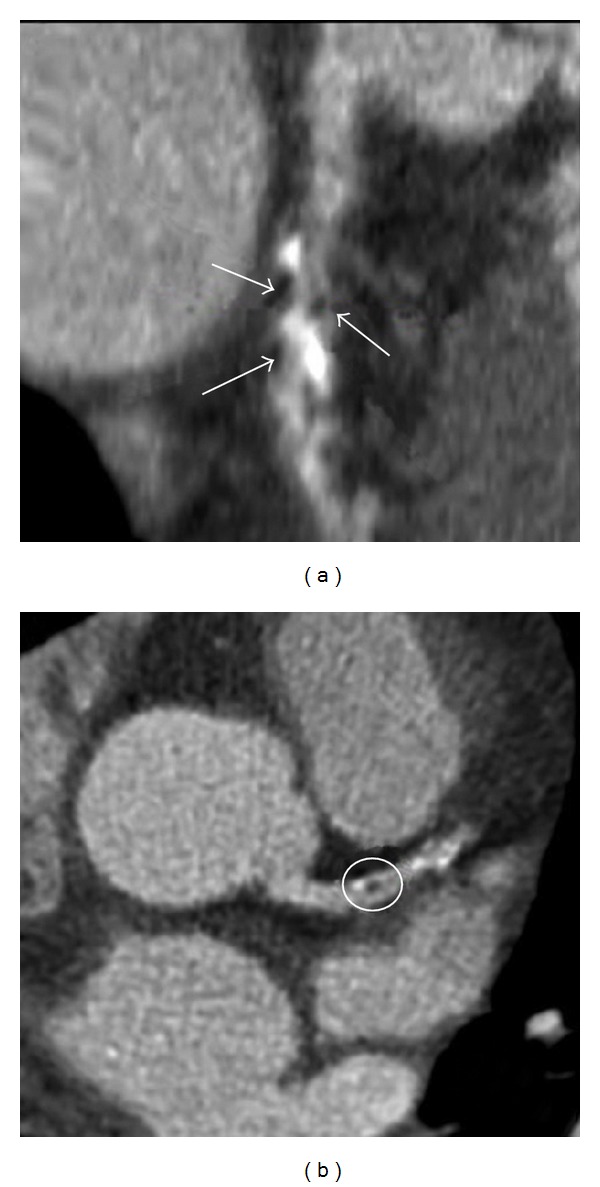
64-slice coronary CT angiography (CCTA) in a 57-year-old man with non-ST elevation myocardial infarction. (a) CCTA image of multiplanar reconstruction of left anterior descending artery (LAD) showing multiple black low-density areas (white arrows) within the plaque. (b) Visualization of the LAD stenosis showing positive remodelling and presence of low-density plaque (<30 Hounsfield units) in the middle of the plaque (black area, white circle). Reprint with permission from [24].
